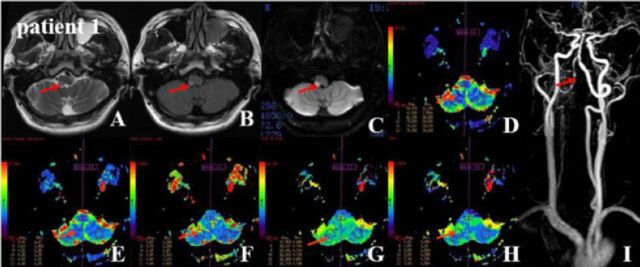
Magnetic resonance imaging-T2 weighted imaging, fluid attenuated inversion recovery (Flair) and diffusion weighted imaging showed a-c) high signal intensities in the right lateral medulla, d-h) Dynamic susceptibility contrast-enhanced-perfusion weighted imaging demonstrated a lesion ROI high cerebral blood flow, high cerebral blood flow, delayed mean transit time, decreased time to peak, and decreased time of maximum concentration on the VAH side. The representative rigid quantitative thresholds in the ROIs of 7 and 8 are shown in Table 2. i) constrast-enhanced megnetic resonance artery indicated right VAH and moderate stricture and rosary in the V4 segment of the right vertebral artery and minor kinking of the left vertebral artery. VAH - vertebral artery hypoplasia, ROI - region of interest
